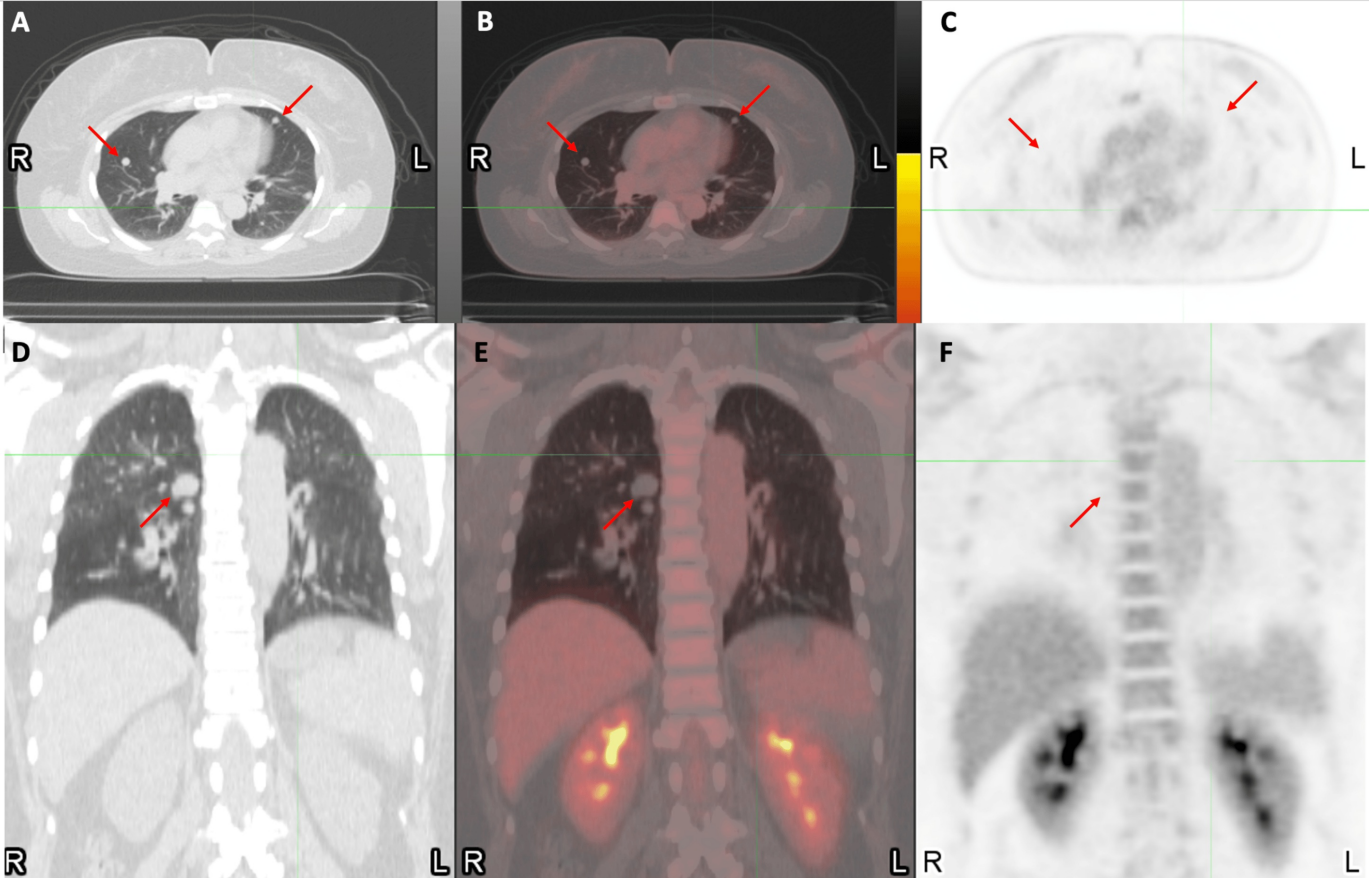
Positron emission tomography imaging of the thoracic cavity demonstrating the lack of hypermetabolism of the pulmonary nodules. Positron emission tomography imaging demonstrates no abnormal fluorodeoxyglucose uptake corresponding to the innumerable pulmonary nodules throughout the bilateral lungs. (a) Axial computed tomography of the thorax. (b) Axial fused positron emission tomography and computed tomography image of the thorax. (c) Axial attenuation corrected image of the thorax. (d) Coronal computed tomography of the thorax. (e) Coronal fused positron emission tomography and computed tomography image of the thorax. (f) Coronal attenuation corrected image of the thorax. Representative pulmonary nodule locations are indicated using red arrows.
